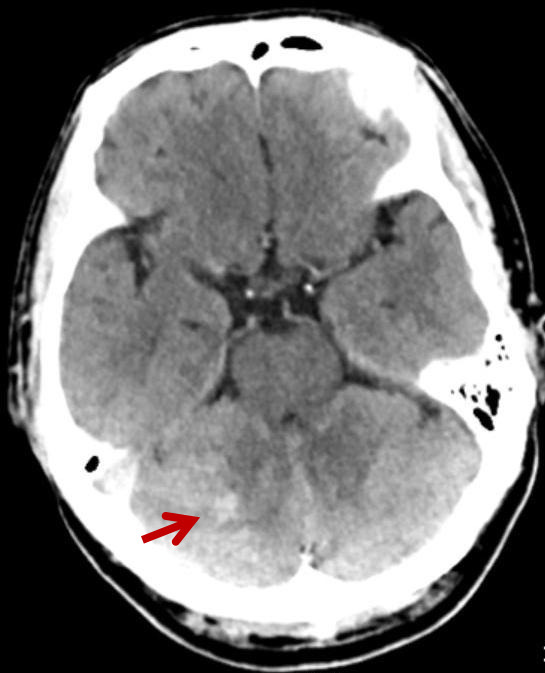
Emergency non-contrast CT showed multiple hyperdensities in the cerebellum (arrow).
